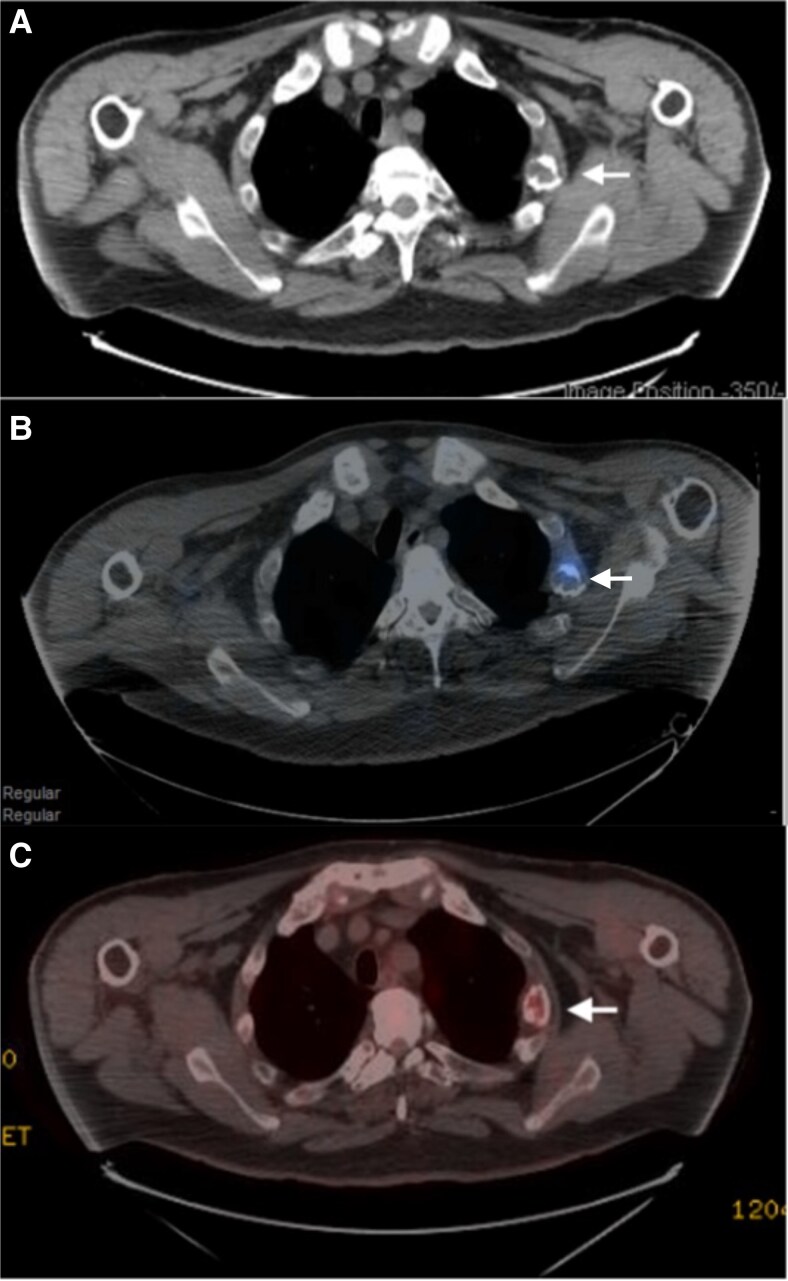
Anatomical and fused functional imaging studies. A, Computed tomography (CT) of the chest showed an expansile lytic lesion with soft tissue component associated with a healing callus on the left lateral third rib. B, Fused octreotide single photon emission (SPECT)/CT showed increased uptake in the lytic lesion on the left third rib. C, ^18^F-fluorodeoxyglucose (^18^F-FDG) positron emission tomography (PET)/CT also showed increased uptake in the same area, concordant with the octreotide scan, suspicious for a phosphaturic mesenchymal tumor.
